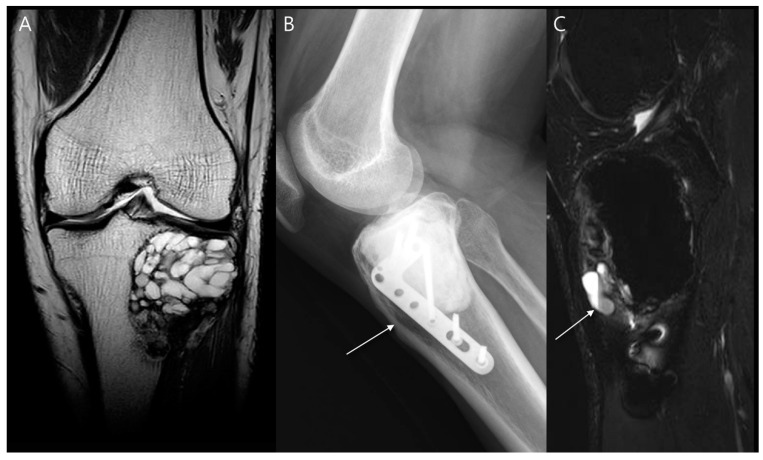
Local recurrence after intralesional curettage with cementation for giant cell tumor of bone. (A) Preoperative coronal T2-weighted MR image shows a well-defined osseous lesion with aneurysmal bone cyst changes in the proximal tibia. (B) One year later, a radiograph shows deep periprosthetic lucency anterior to the cement insertion (arrow), raising suspicion of recurrence. (C) Sagittal T2-weighted fat-suppressed MR image confirms the presence of a recurrent lesion with aneurysmal bone cyst changes (arrow).
